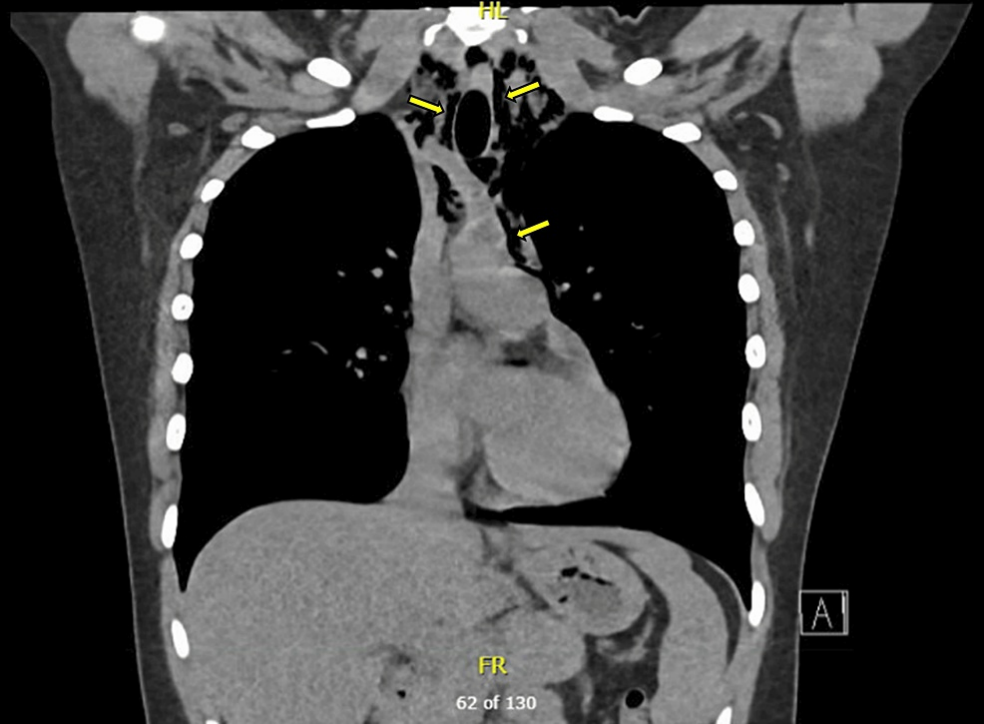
CT chest of a PM with subcutaneous emphysema extending into the neck Coronal CT chest with a mediastinal window showing PM with subcutaneous emphysema extending into the neck. Arrows denote free air accumulation within the mediastinal space, reaching the thoracic outlet and surrounding the paratracheal neck space CT: computed tomography; PM: pneumomediastinum
